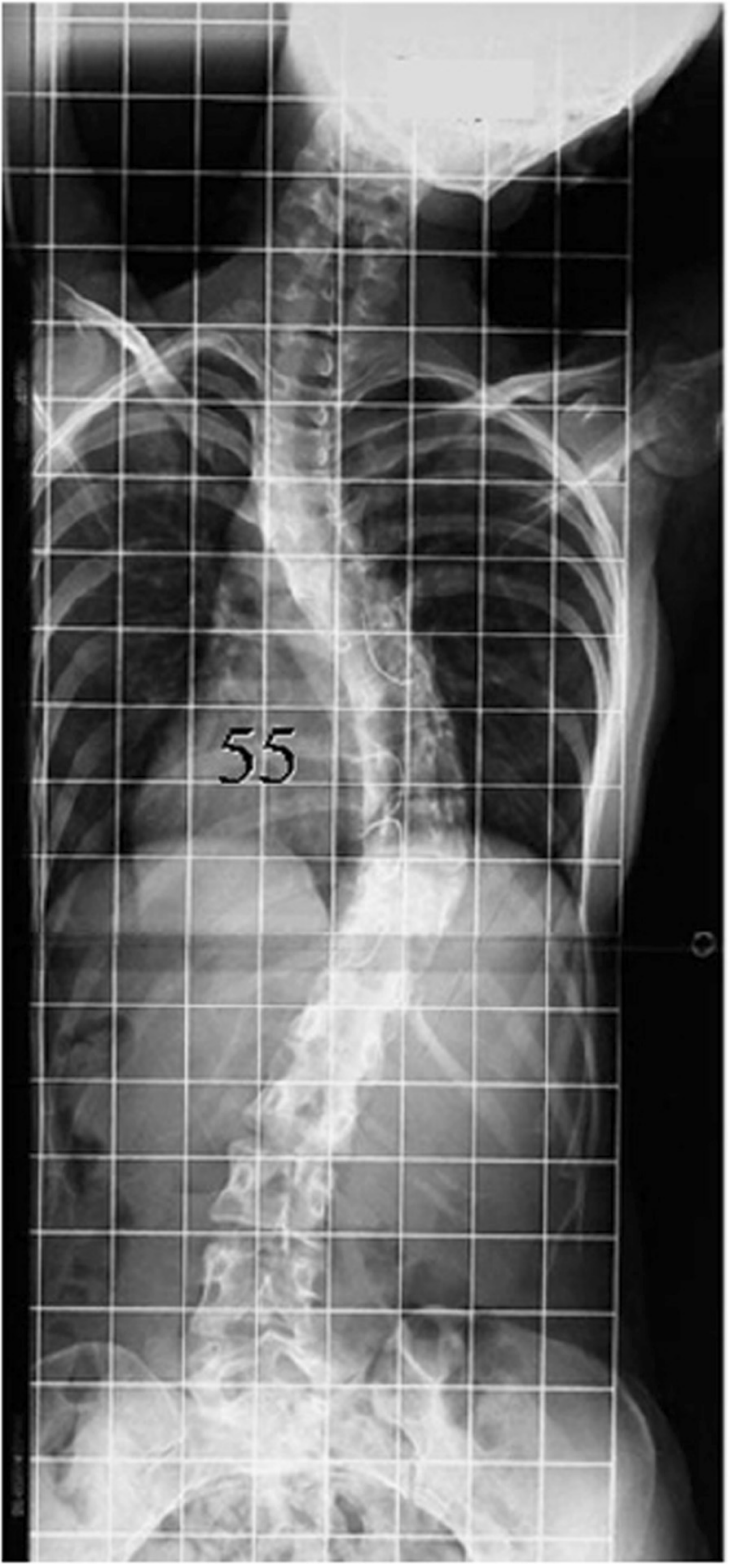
Bending radiograph prior to reinstrumentation.
